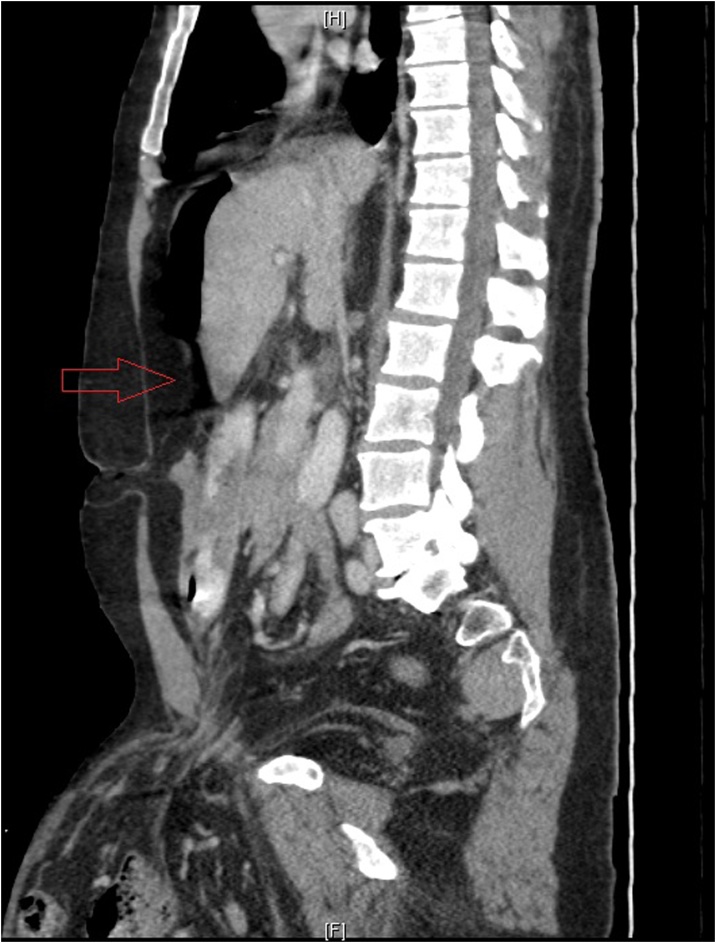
CT scan of the abdomen. free air (arrow).
